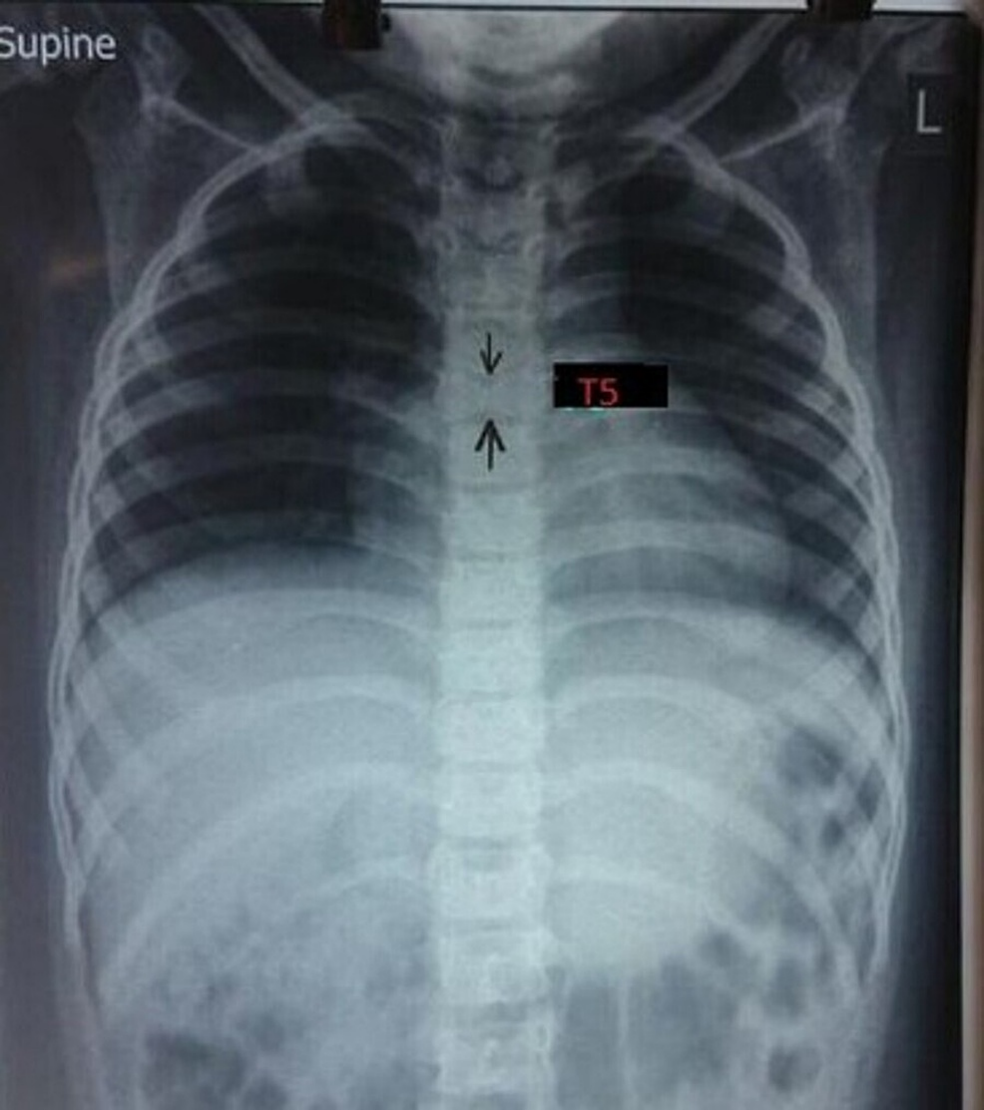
Chest X-ray showing butterfly presentation of the T5 vertebra.
